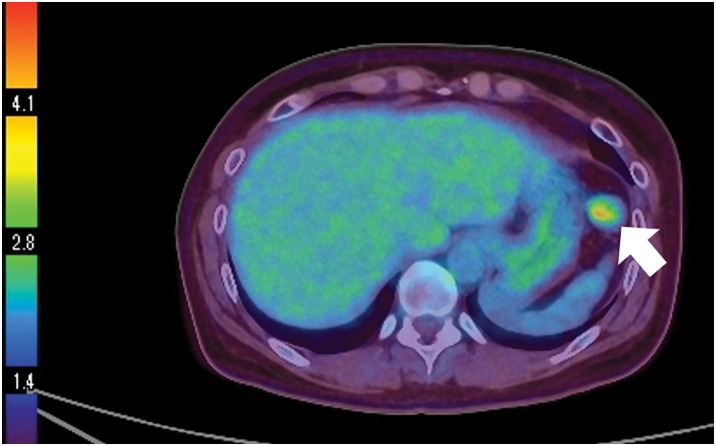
FDG-PET/CT. Abnormal accumulation of SUVmax 4.2 was observed in the mass observed on plain CT.
